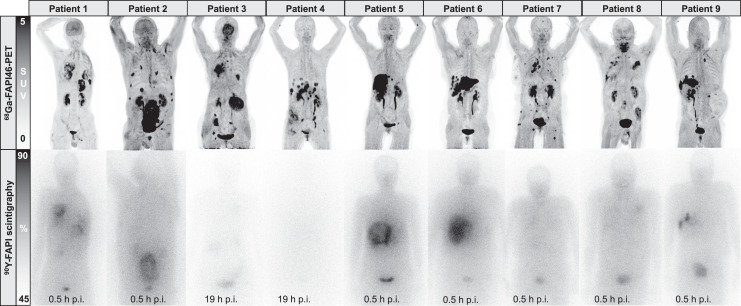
Pretreatment ^68^Ga-FAPI-46 PET images and posttreatment ^90^Y-FAPI-46 bremsstrahlung scintigraphs after first cycle of ^90^Y-FAPI-46 RLT. p.i. = after injection.
